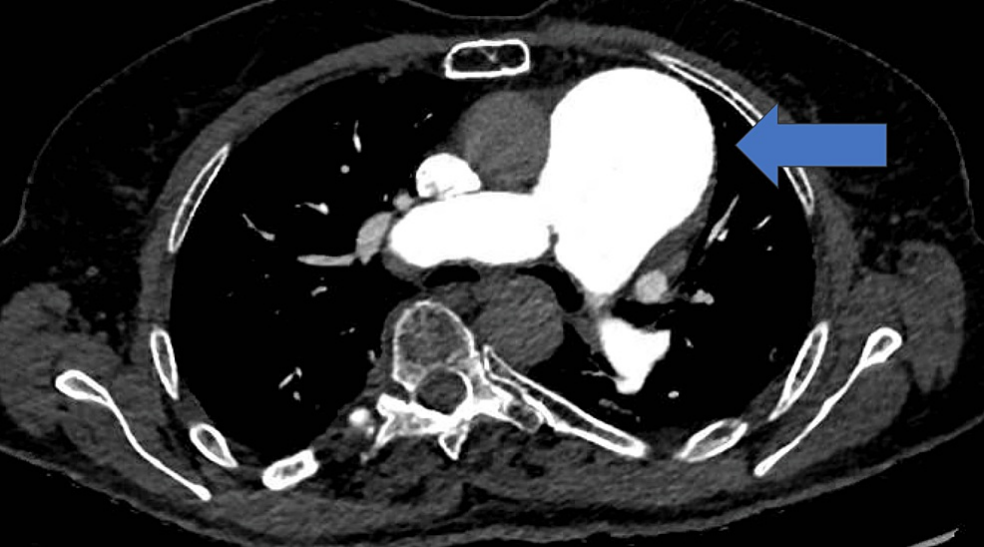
Axial plane of pre-operative CT scan showing a giant PAA PAA: Pulmonary artery aneurysm
